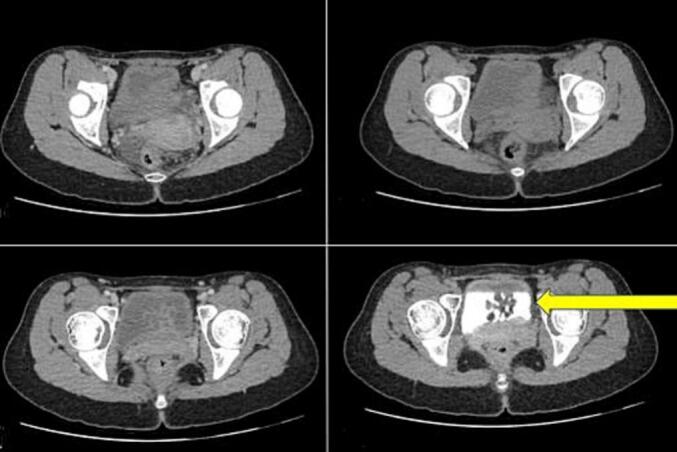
Sequential CT scan images polypoidal lesions arising from the base of urinary bladder and projecting into the lumen.
